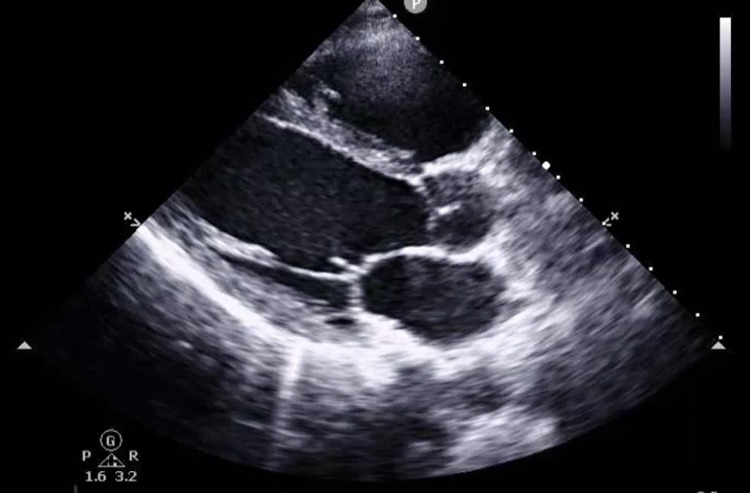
Parasternal long axis trans thoracic echocardiographic image
